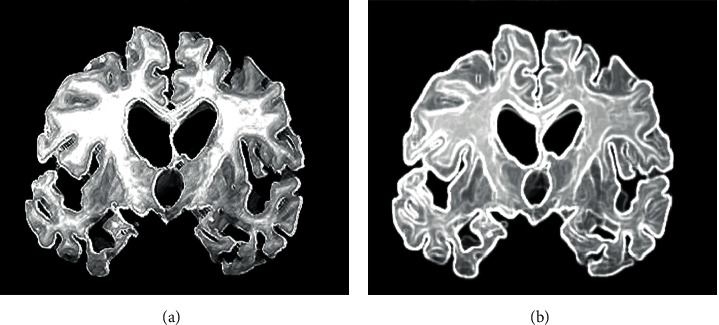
MRI images of AD patients processed by CNN and DML algorithms. *Note.* Figure 4A was an MRI image of AD patients based on CNN algorithm; and Figure 4B was an MRI image of AD patients based on DML algorithm.
